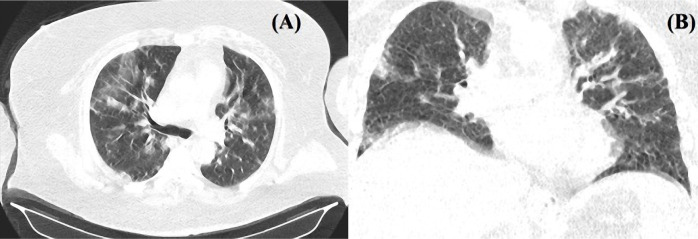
Chest CT. Axial cross-section (A) and coronal cross-section (B) showing diffuse areas with ground-glass attenuation.
